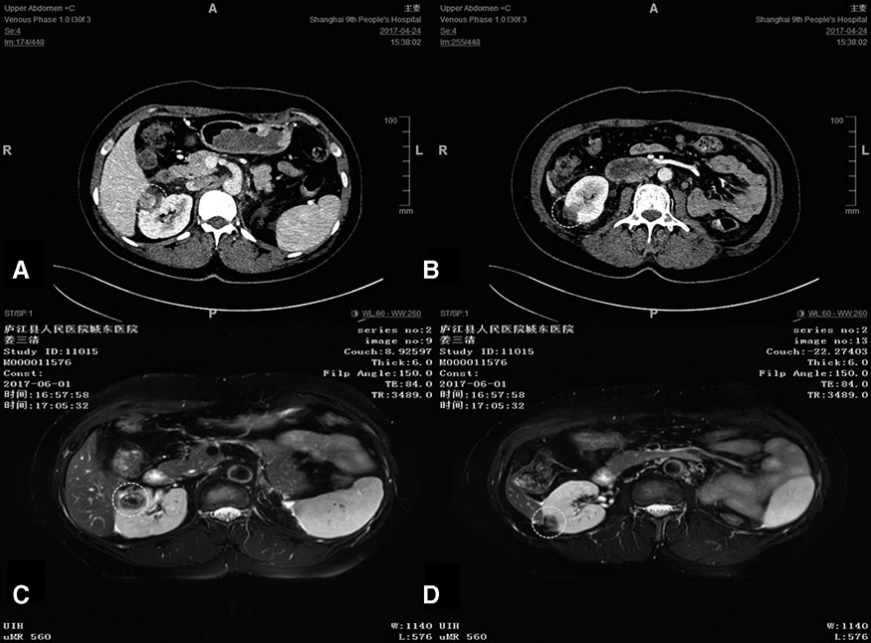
Contrast-enhanced CT scan 4 days after surgery, and contrast-enhanced MRI scan of the kidney 6 weeks after surgery. (A) The mass in upper pole of the right kidney 4 days after surgery. (B) The mass in the middle pole of the right kidney 4 days after surgery. (C) The mass in the upper pole of the right kidney 6 weeks after surgery. (D) The mass in the middle pole of the right kidney 6 weeks after surgery. The *dotted circles* indicate the ablation part of the tumor after surgery.
